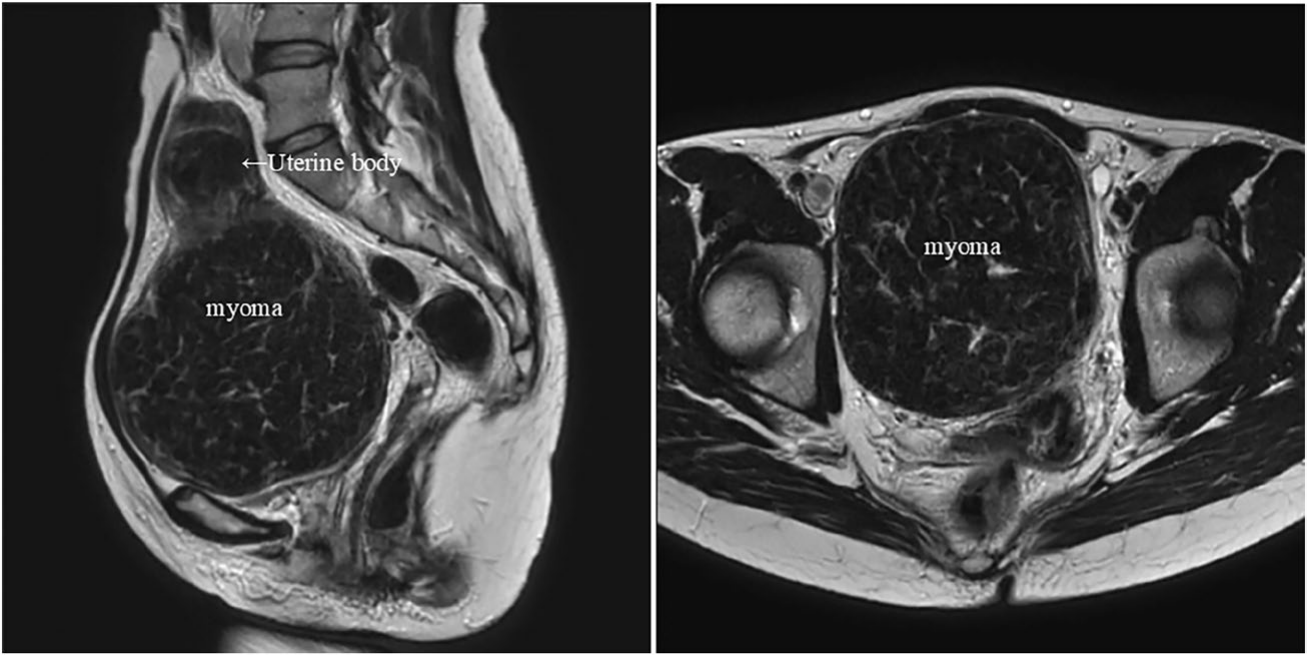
Magnetic resonance imaging scan of the 120‐mm cervical myoma and other myomas. Sagittal view of the uterine body (left) and axial view of the uterine myoma (right).
